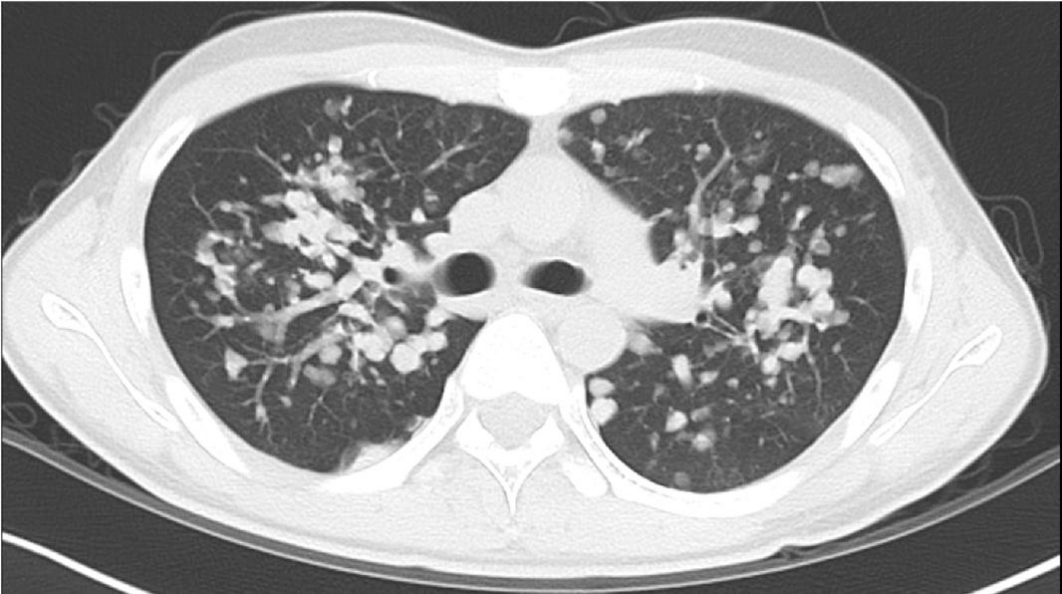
Chest tomography (CT) findings in a Japanese patient with acquired immunodeficiency syndrome (AIDS) and pulmonary infection caused by *Trametes elegans* and *Peniophora incarnata* in a study of emerging fungal infections. Multiple well-defined nodules are diffusely distributed throughout both lungs, with central predominance. Some lesions were also observed in the subpleural regions.
